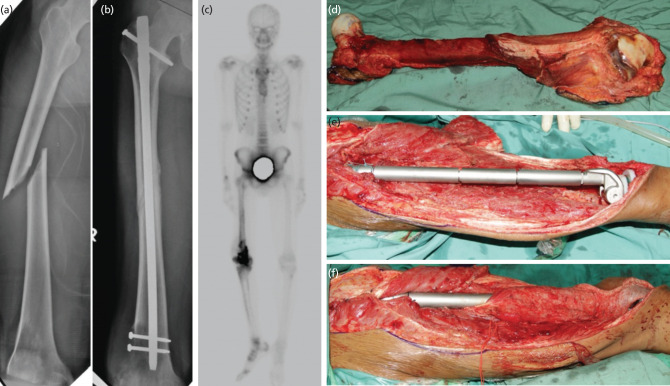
Case 7. A 23-year-old gentleman presented with a traumatic spiral fracture involving the midshaft of the right femur. Radiograph revealed the presence of an ill-defined osteolytic lesion in the distal metaphysis of the ipsilateral femur associated with cortical destruction with minimal soft tissue opacity at the posterolateral aspect of the region. He was initially treated with an interlocking nail, and the fracture united. However, there was a progression of distal femur lytic lesion, and biopsy confirmed the conventional type of osteosarcoma. He underwent four courses of chemotherapy and responded well. Total femur with distal quadriceps resection was performed to achieve a wide and adequate margin due to contamination from previous surgery. The limb was reconstructed with total femur endoprosthesis and a motorised latissimus dorsi flap. He is now 10 years post-surgery, disease-free, and ambulating without support with a 20° knee extension lag due to quadriceps weakness. (a) Traumatic oblique fracture mid-shaft femur with early lytic and rarefaction of distal femur. (b) the distal femur lytic lesion progressed but the fracture united well. (c) Bone scan showed active lesion occupied the distal femur and no evidence of distant metastases. (d, e, f) resection of the entire femur with anticipated contamination to the soft tissue and endoprosthesis replacement with latissimus dorsi flap.
